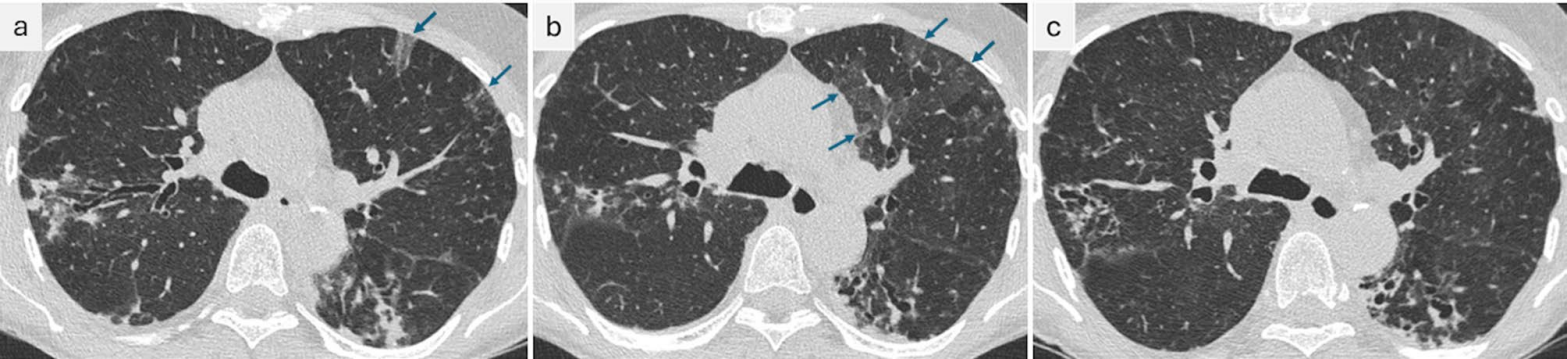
Thoracic CT scans (axial plane, 1-mm-slices). a. CT scan at 3 months of ALIS-based antimycobacterial treatment in 06/2022: decreasing consolidations compared to Fig. 1c. Focal ground glass opacities (GGO) in the left upper lobe (arrows). b. CT scan after completion of antimicrobial treatment in 05/2023. Increased GGO in the left upper lobe (arrows) c. Decrease of GGO at 7 months after completion of ALIS treatment
